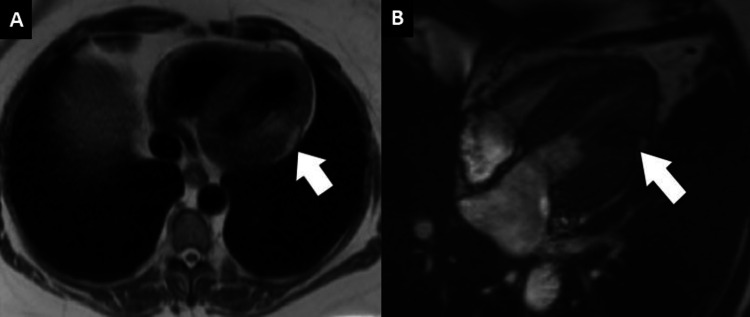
Gadolinium-enhanced cardiac MRI (A) T1-weighted sequence (axial view) showing regions of increased signal intensity (fat-containing) in the epicardial mid-lateral wall and base of the anterolateral papillary muscle. (B) T2-weighted sequence (axial view) showing decreased signal intensity in the same area.
